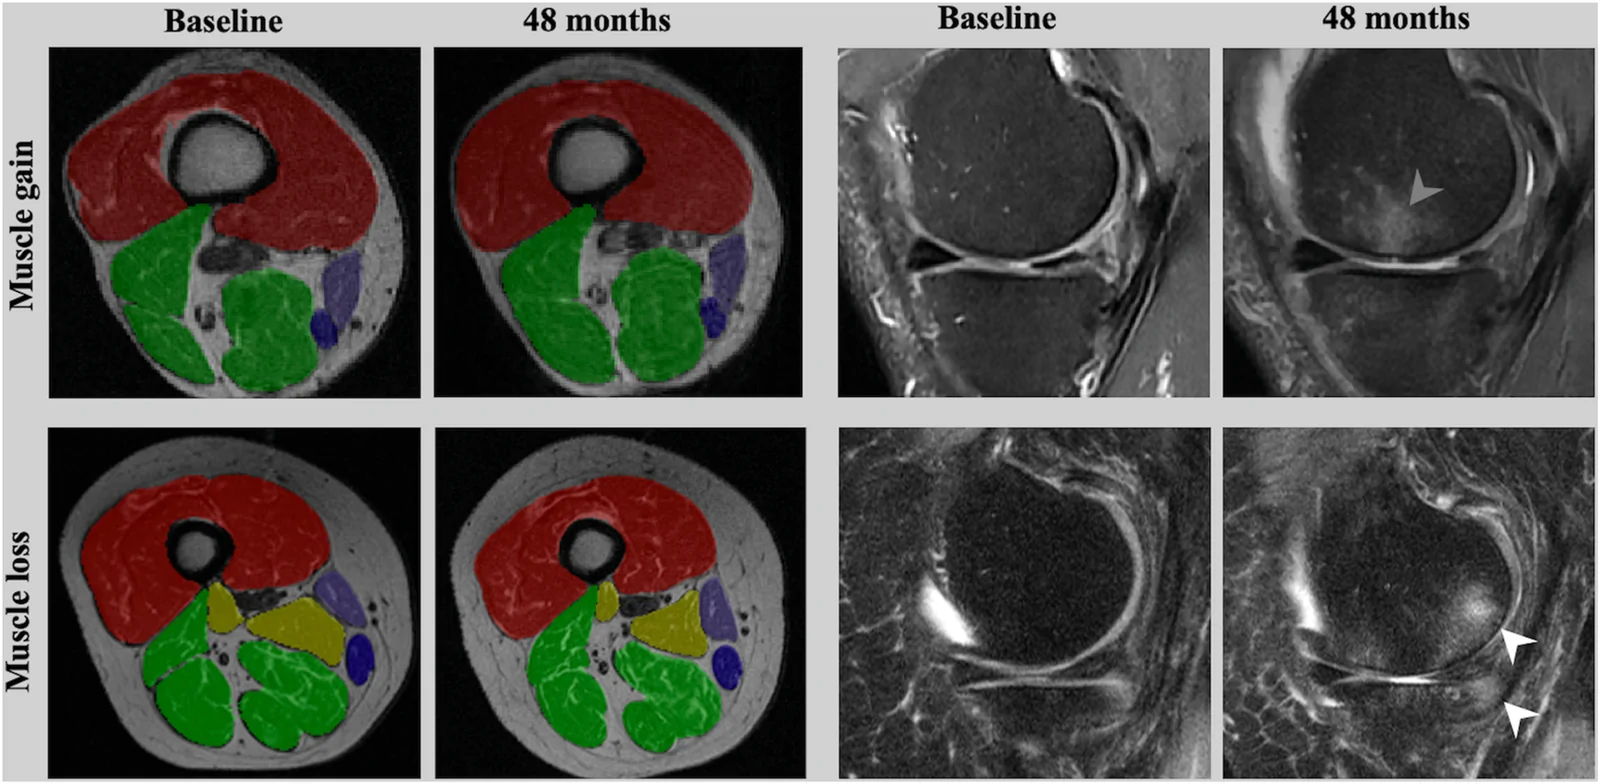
Imaging examples of changes in bone marrow edema-like lesions (BMELL). Left: Axial T1-weighted MR images of the right thigh with artificial intelligence-enabled muscle segmentation at baseline and after 48 months. Right: Sagittal intermediate-weighted fast spin-echo fat-suppression sequence of the right knee at baseline and after 48 months. The patient with muscle gain (predominantly in the quadriceps muscle, indicated in red, with an approximately 11% increase in total muscle volume over 48 months) was a 65-year-old man with mild knee pain at baseline (WOMAC pain subscale of 1) and no knee pain at 48 months (WOMAC pain subscale of 0). Despite the clinical improvement, new BMELLs developed in the medial femoral condyle (grey arrowhead), with baseline WORMS BMELL grades of 0 and 1 for medial femoral condyle at 48 months. The patient with muscle loss (predominantly in the quadriceps muscle (red) and hamstrings (green) with an approximately 14% decrease in total muscle volume over 48 months) was a 64-year-old woman with mild knee pain at baseline (WOMAC pain subscale of 1) and an increase of knee pain at 48 months (WOMAC pain subscale of 11). New BMELL developed in the medial tibial plateau and in the medial femoral condyle (white arrowheads), with baseline WORMS BMELL grades of 0 and 3 for both medial tibial plateau and medial femoral condyle at 48 months.
